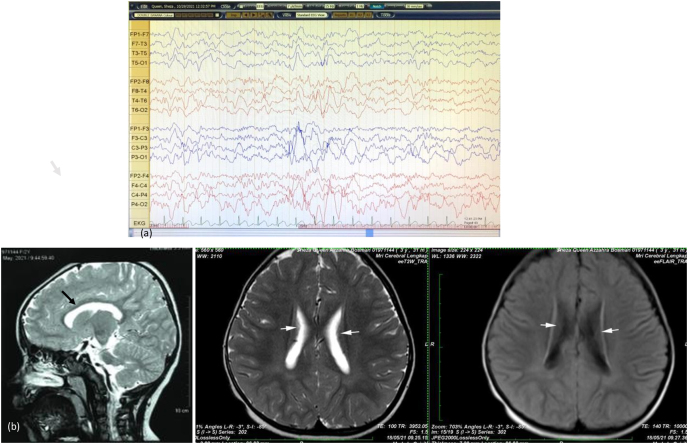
(a) EEG recording showing abnormal pattern during sleep with medium amplitude rhythmic 2-3c/s. (b) Sagittal and axial brain MRI showed microcephaly, corpus callosum dysgenesis, and heterotopia grey matter on the bilateral lateral ventricle.
